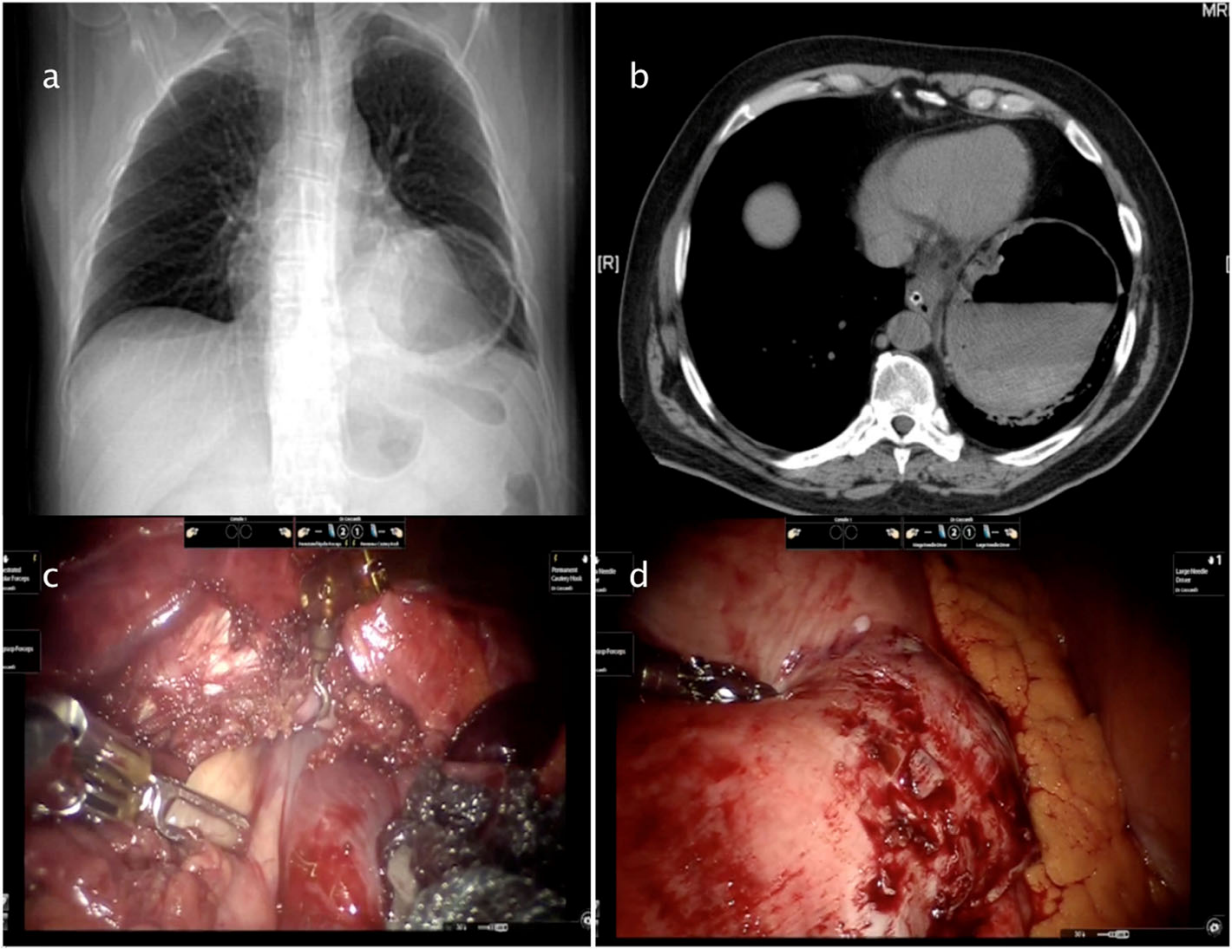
Case 2: Preoperative radiologic exams and intraoperative images. a–b Chest radiography and chest-abdomen CT scan showing an air-fluid level at the posterior mediastinum. c–d Gastric reduction after hiatal defect enlargement
